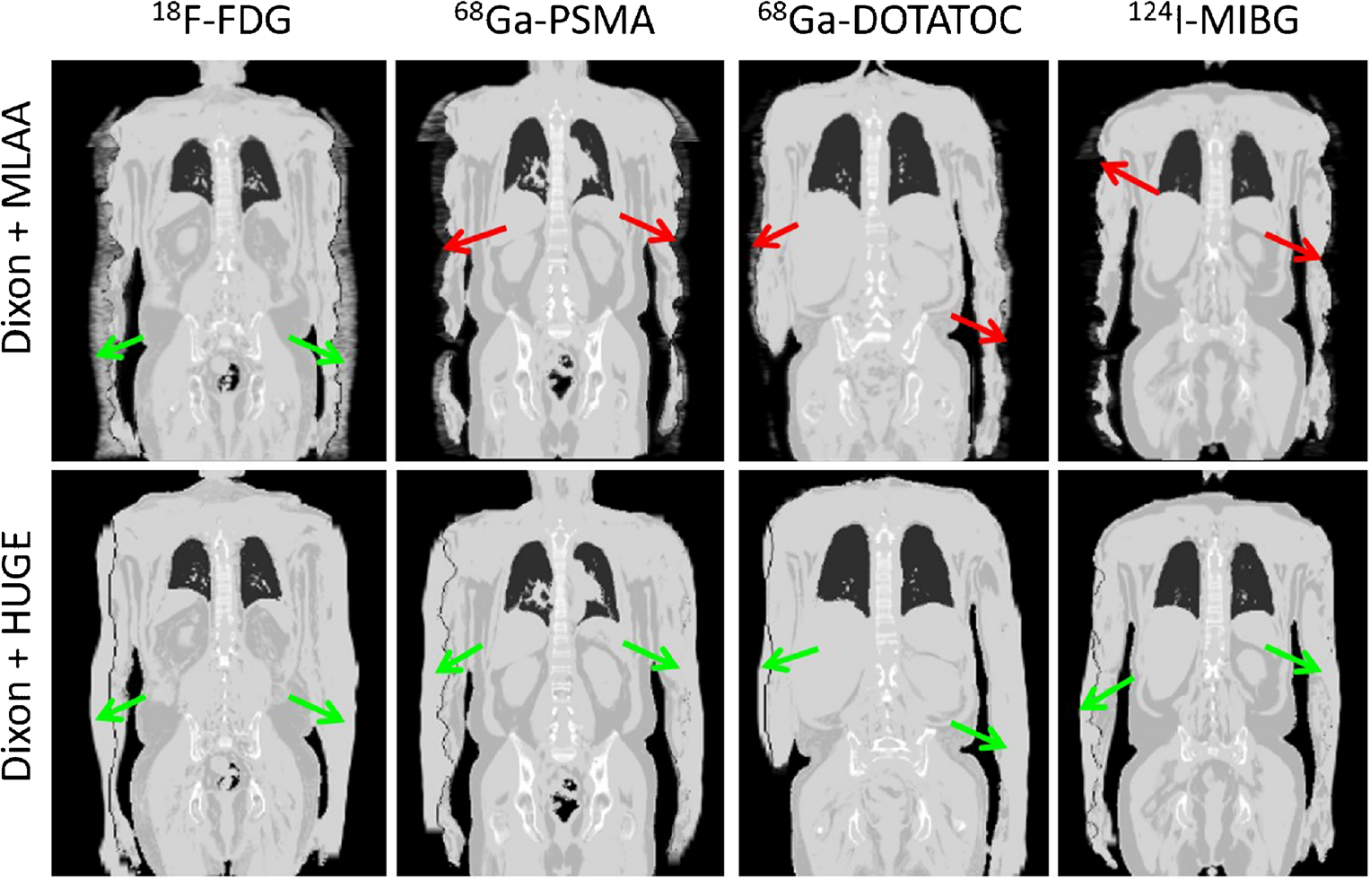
μ-maps with applied truncation correction due to PET-based MLAA (upper row) or MR-based HUGE (lower row) in four patient examples using four different radiotracers (^18^F-FDG, ^68^Ga-PSMA, ^68^Ga-DOTATOC, ^124^I-MIBG). Green arrows indicate accurate truncation correction, whereas red arrows indicate incomplete or failed truncation correction. Note that the MR-based HUGE method works robust, independent of the choice of radiotracer. MLAA provided acceptable truncation correction only in the patient example with ^18^F-FDG
